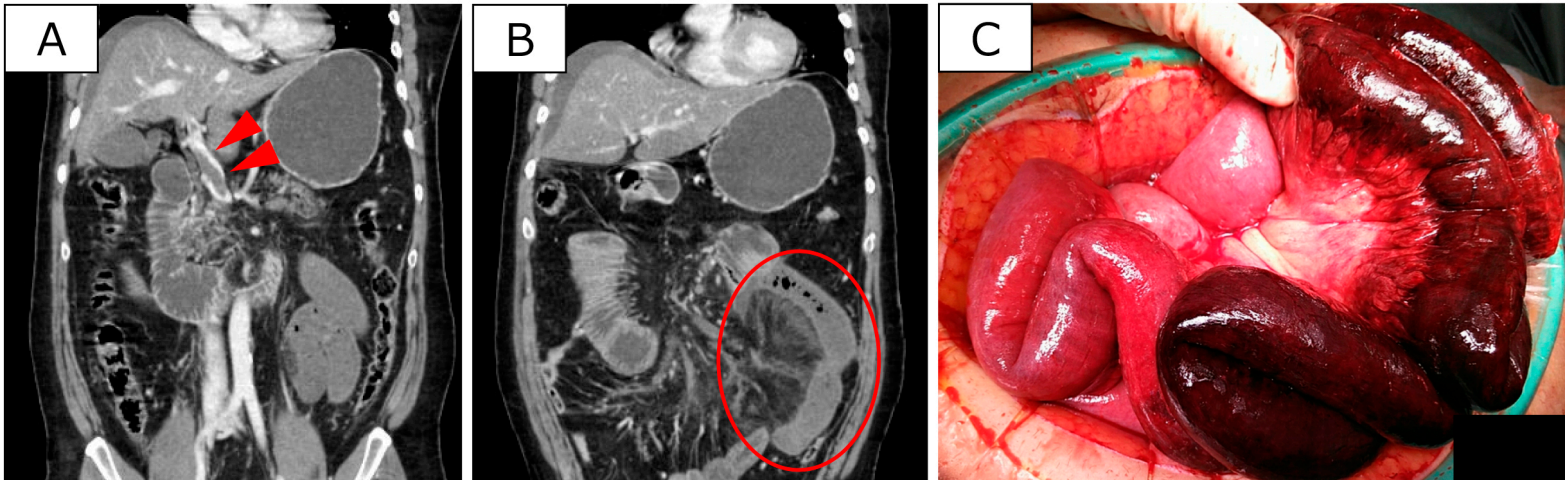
Contrast-enhanced computed tomography (CT) images upon arrival and intraoperative findings. (A) Coronal view showing an extensive thrombus in the main trunk of the portal vein (arrowhead). (B) A poor contrast area within a section of the small intestine accompanied by intestinal wall edema and thickening (circles). (C) Intraoperative findings showing bloody ascites, extensive necrosis of approximately 150 cm of the jejunum, and mesenteric edema.
